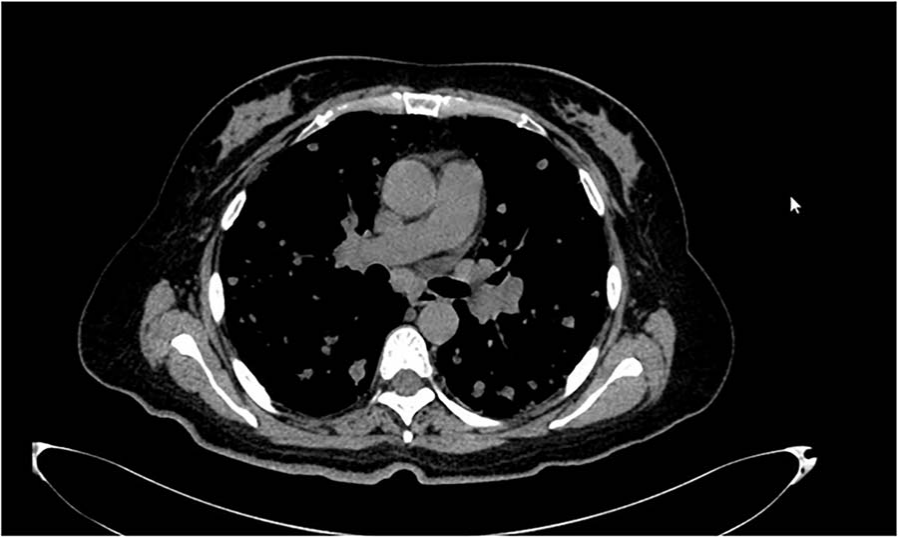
Axial thoracic CT scan showing multiple bilateral pulmonary nodules in both lower lobes, highly suggestive of hematogenous metastatic spread from gestational choriocarcinoma.
